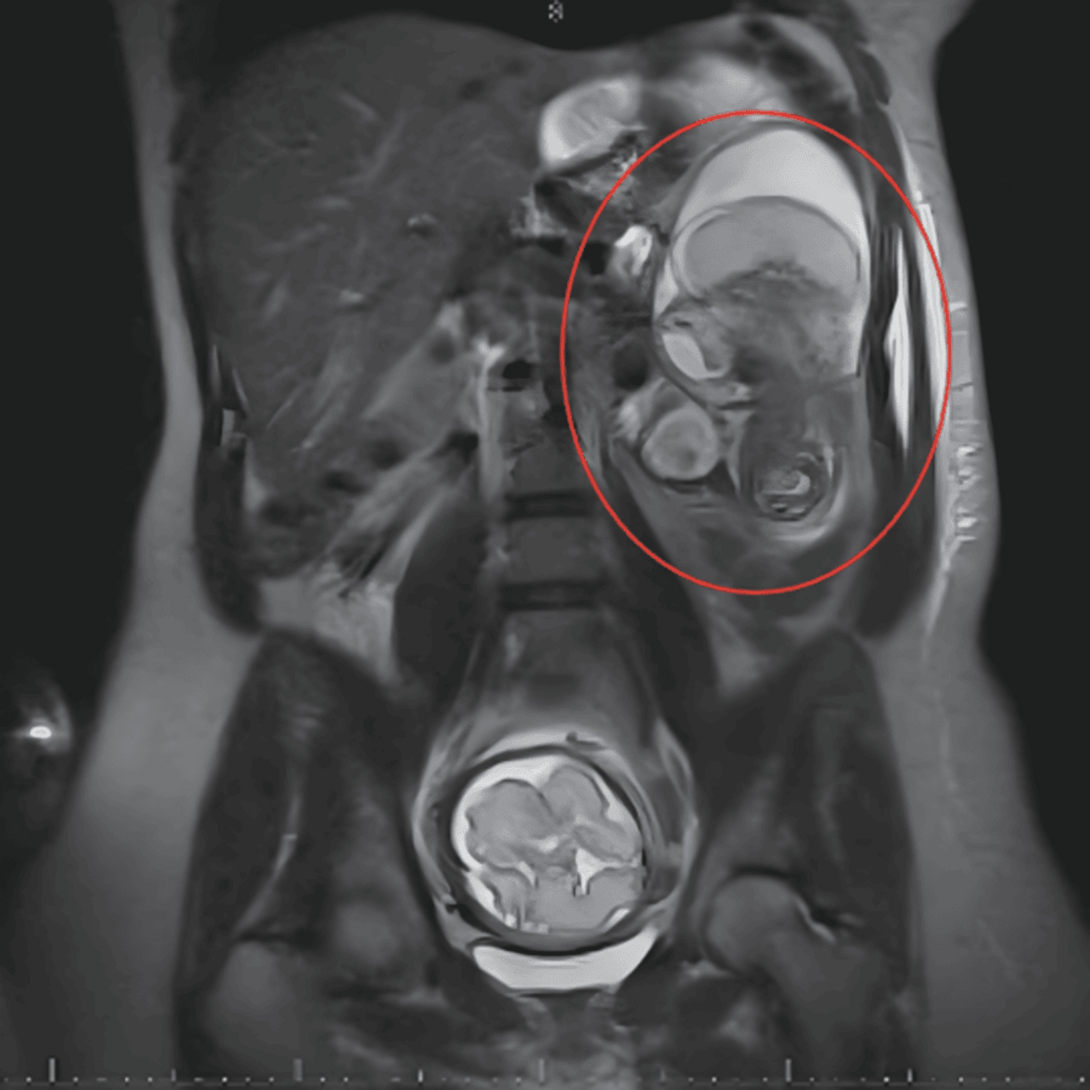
Abdominal MRI Magnetic resonance imaging (MRI) of the abdomen/pelvis of the 37-year-old female who was 29 weeks pregnant with symptoms consistent with a small bowel obstruction. Official read identified a closed-loop intestinal dilation suggesting an incarcerated internal hernia in the presence of a previous Roux-en-Y gastric bypass, suggesting a Petersen’s hernia.
